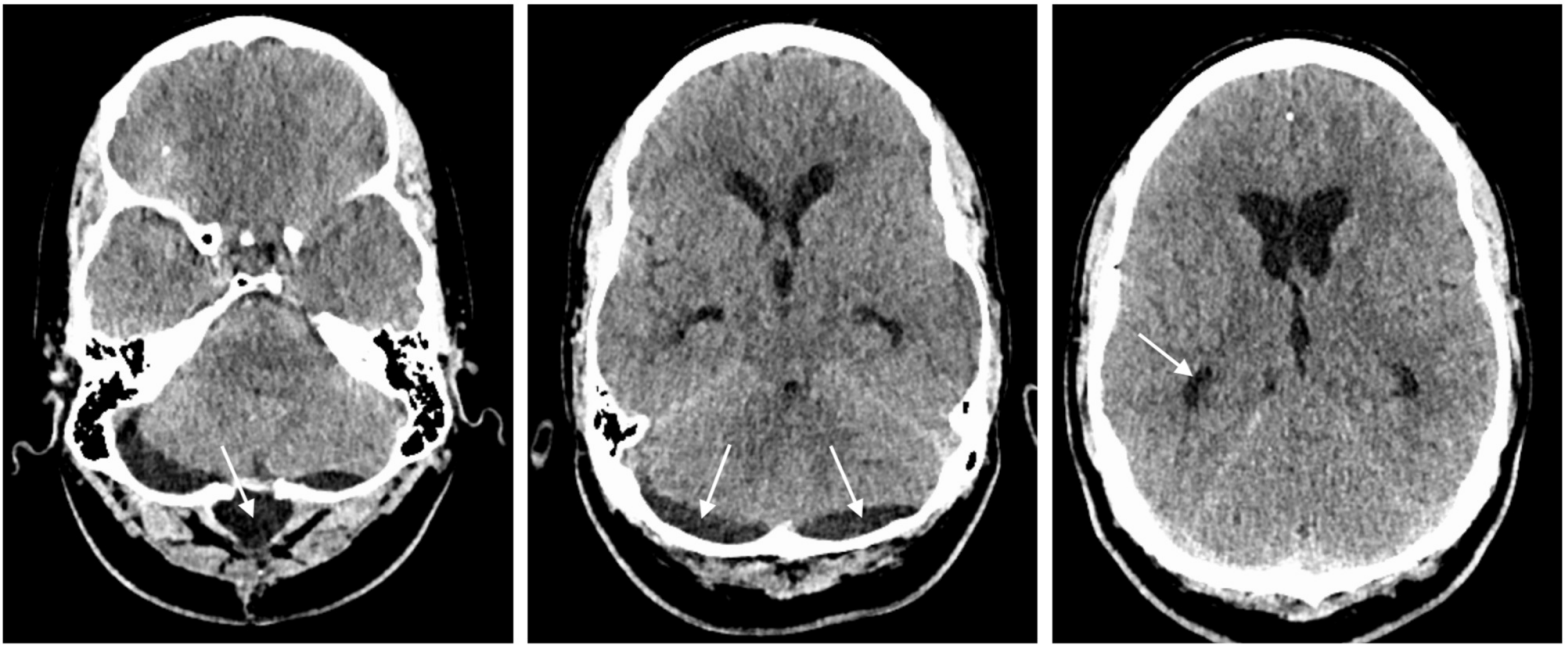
CT head two months following foramen magnum decompression. CT head demonstrating small pseudomeningocele in relation to foramen magnum decompression (arrow in left image), bilateral posterior fossa subdural hygromas (arrows in middle image) and mild hydrocephalus with dilated temporal horns (arrow in right image).
